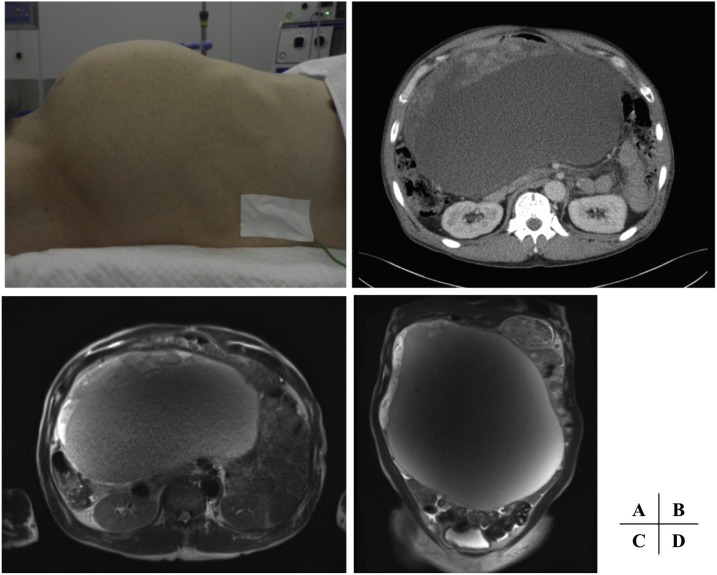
A. Distended abdomen before surgery. B. Computed tomography revealed the large cyst and solid component showing enhancement. C, D. T2-weighted magnetic resonance imaging also showed the large cyst and solid component with high signal intensity.
